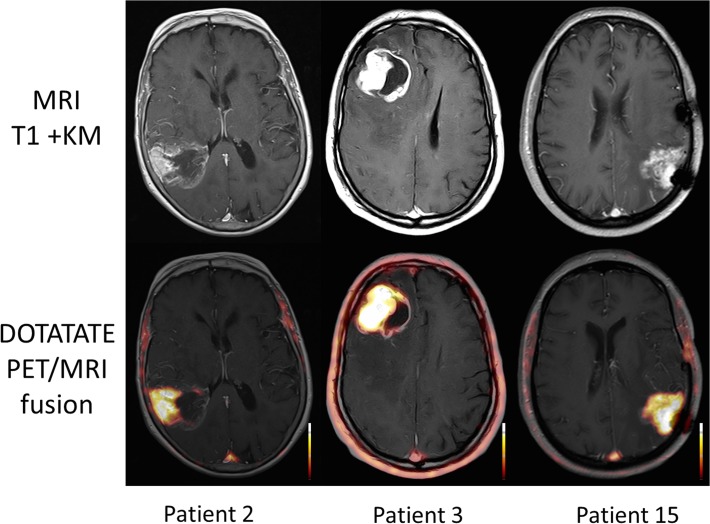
Comparison of ^68^Ga-DOTATATE uptake and MR contrast agent enhancement. In all 3 patients, there is a marked overlap between ^68^Ga-DOTATATE uptake and MR contrast agent enhancement. Radiotracer accumulation is most pronounced in areas with intense contrast enhancement. Shown are axial views of contrast-enhanced T1-weighted MR as well as fused MR/PET images.
